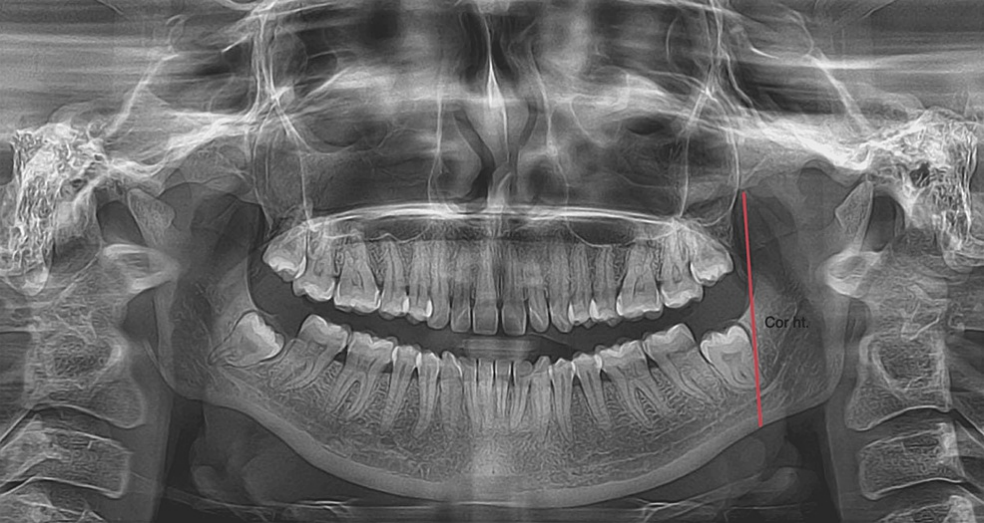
An orthopantomogram represents the coronoid ramus height (Cor. ht) of the mandible
